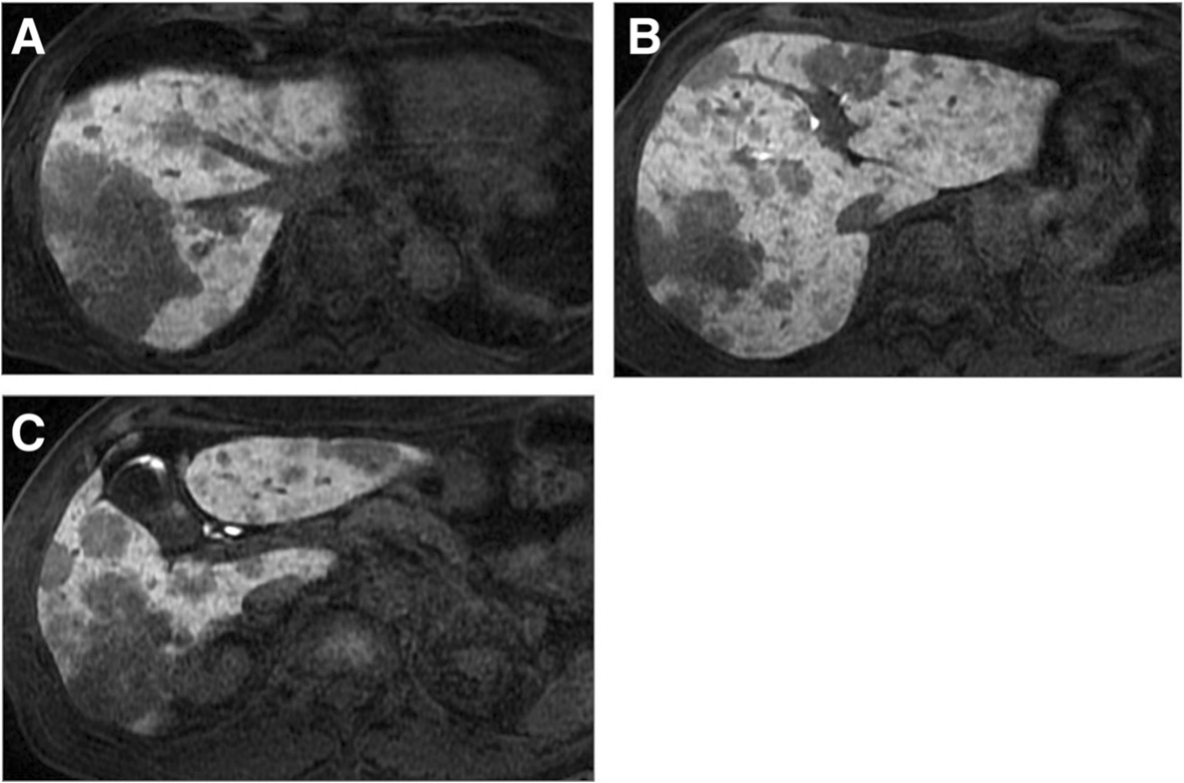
Abdominal contrast-enhanced magnetic resonance imaging before the second tumor biopsy. a–c Some tumors show heterointensity, and other tumors show central enhancement in the parenchymal phase of T1-weighted image
